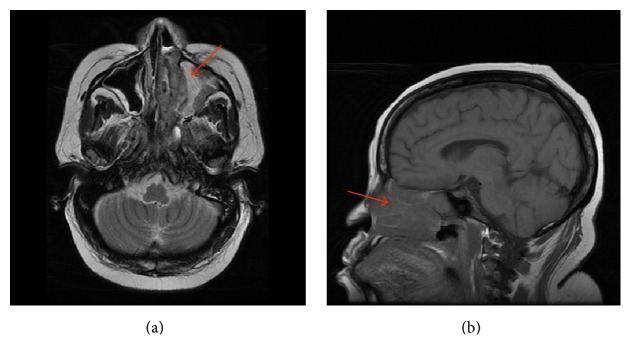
((a) (axial) and (b) (sagittal)) MRI of the brain showing a soft tissue mass in the left maxillary sinus and left nasal cavity with extension to involve the medical left orbit.
